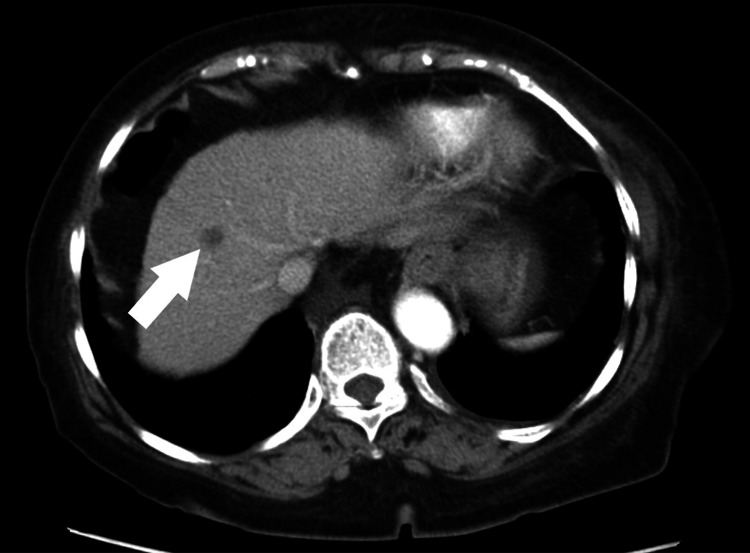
Axial view of the abdominal contrast-enhanced CT scan (arterial phase) One small hypovascular tumor suspected of liver metastasis was confirmed in the liver (arrow).
